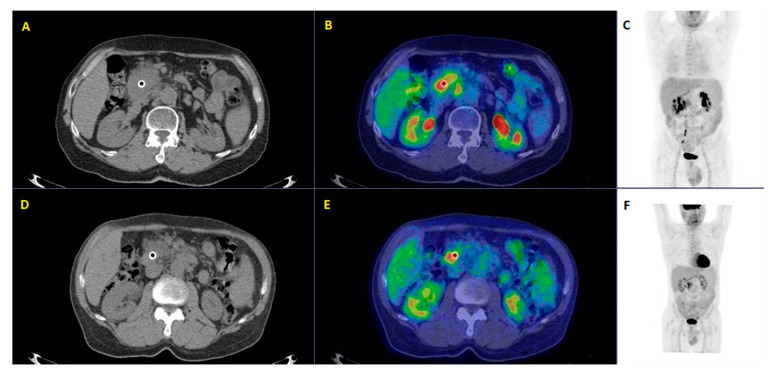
A fifty-four-year-old patient affected by local invasive ductal adenocarcinoma underwent restaging ^18^F-FDG PET/CT before and after chemotherapy. ^18^F-FDG PET/CT after 4 cycles of FOLFIRINOX (A–C) compared to the same examination before chemotherapy (D–F) revealed increasing metabolic activity of the pancreatic lesion (SUVmax = 14 vs. 10) and the appearance of a new locoregional lymph-nodal uptake in the surrounding fat (SUVmax = 7), demonstrating the lack of therapy response with the subsequent modification of therapy management. (Images from Nuclear Medicine Unit, Fondazione Istituto G.Giglio of Cefalù, Italy). Legend: A = Axial image of low-dose CT after chemotherapy; B = PET/CT fused image after chemotherapy; C = Maximum intensity Projection (MIP) after chemotherapy; D = Axial image of low-dose CT before chemotherapy; E = PET/CT fused image before chemotherapy; F = Maximum intensity Projection (MIP) before chemotherapy.
